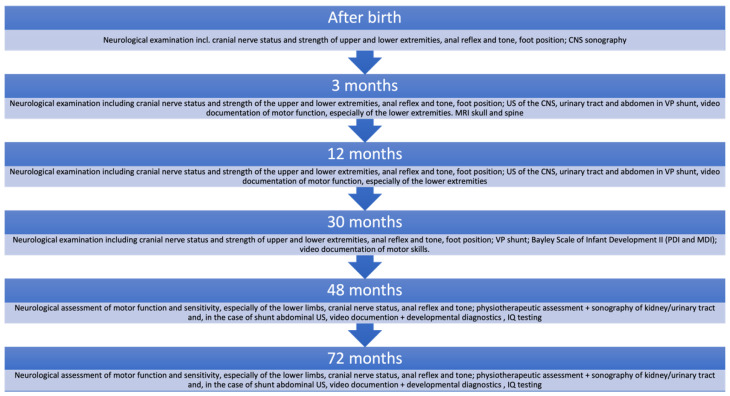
UKGM’s own long-term follow-up: parents are asked to attend regular neuropediatric checkups, MRI imaging is dependent on the child’s clinical condition, but at least once a year. Children are followed up until 72 months of age in a standardized protocol.
